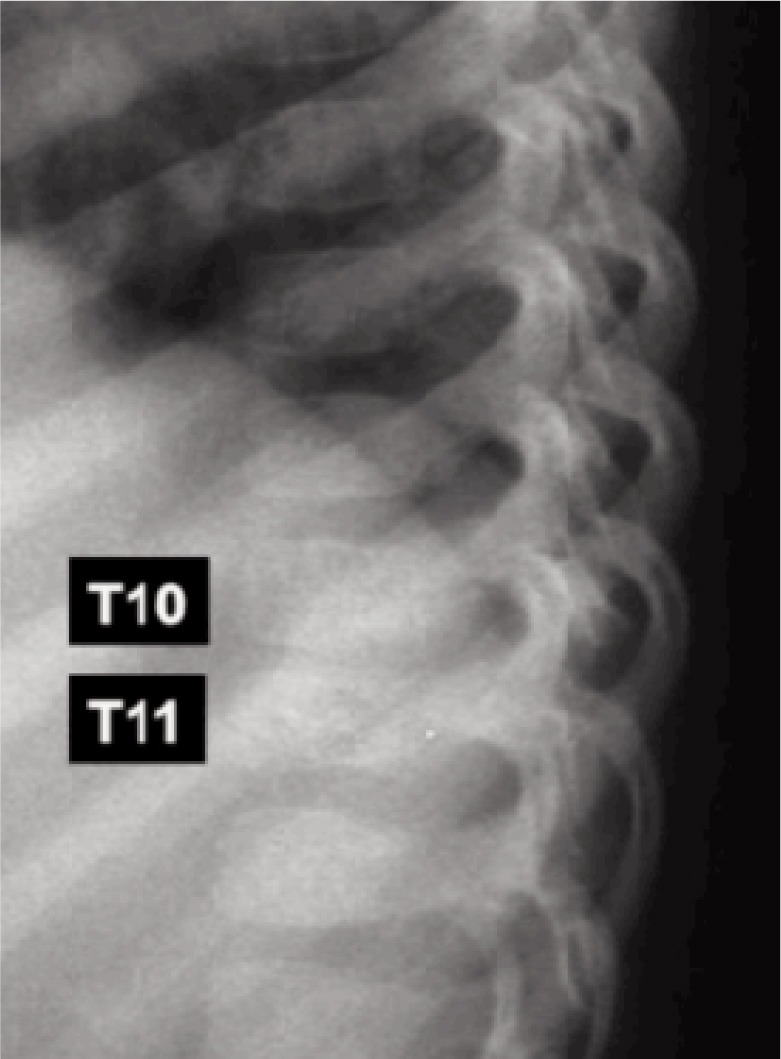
Enlarged lateral radiograph showing reduced T10-T11 disc height.
